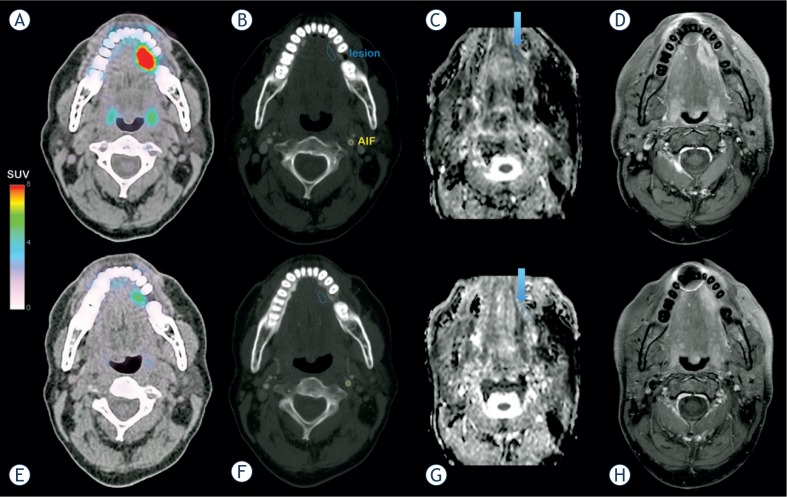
A patient with squamous cell carcinoma of the head and neck. Before cetuximab treatment: (A) PET/CT fusion imaging showing the anatomical location of FDG uptake. (B) CT imaging with the regions of interest used for kinetic analysis. (C) ADC mapping derived from DW-MRI with a measured ADC^lesion^ = 1009 mm^2^.s^−1^ ± 123 mm^2^.s^−1^. (D) Spin Echo T1-weighted MR imaging with fast suppression technique (SPIR) after gadolinium injection (lesion long axis: 2.88 cm, lesion short axis: 1.52 cm). MRI examination was performed 6 days after PET/CT. During treatment with cetuximab: (E) PET/CT fusion showing imaging of the decrease of FDG uptake. (F) CT imaging with the regions of interest used for kinetic analysis. (G) ADC mapping derived from DW-MRI with an increased ADC^lesion^ = 1325 mm^2^.s^−1^ ± 192 mm^2^.s^−1^. (H) Spin Echo T1-weighted MR imaging with fast suppression technique (SPIR) after gadolinium injection (lesion long axis: 2.72 cm, lesion short axis: 1.12 cm). MRI and CT examinations were performed the same day.
